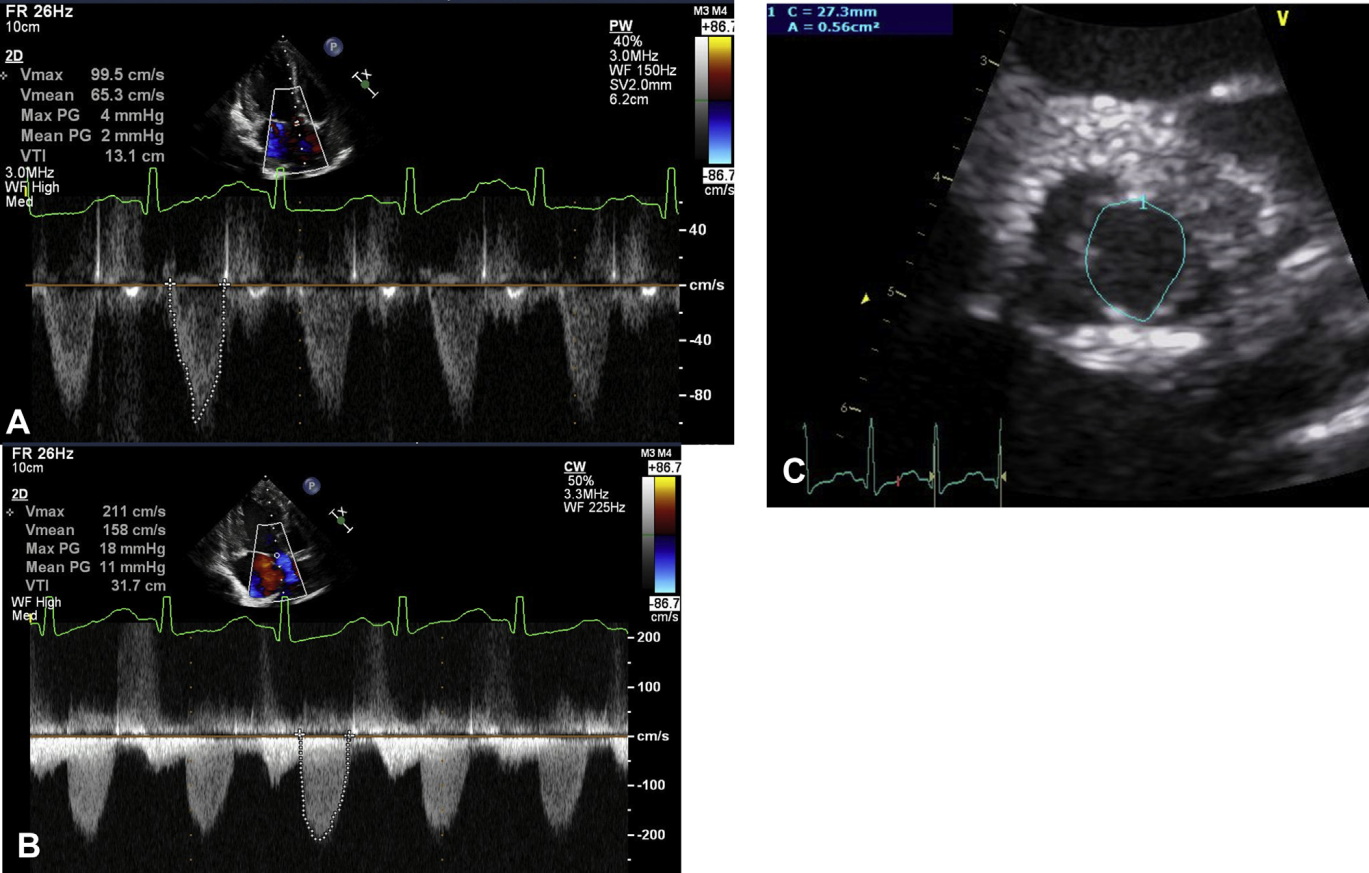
(A) Pulsed-wave Doppler echocardiography performed at the level of the LVOT from the apical 3-chamber window. The velocity-time integral was 13 cm. The calculated stroke volume was 7.2 mL. (B) Continuous-wave Doppler echocardiography across the LVOT and aortic valve from the apical 3-chamber window. The velocity-time integral was 32 cm. The AVA calculated from the continuity equation was 0.21 cm^2^ (0.62 cm^2^/m^2^). (C) Two-dimensional TTE from the left parasternal window shows the aortic valve short-axis view at the midsystolic phase. The traced anatomical AVA calculated from 2D TTE planimetry was 0.56 cm^2^ (1.70 cm^2^/m^2^).
